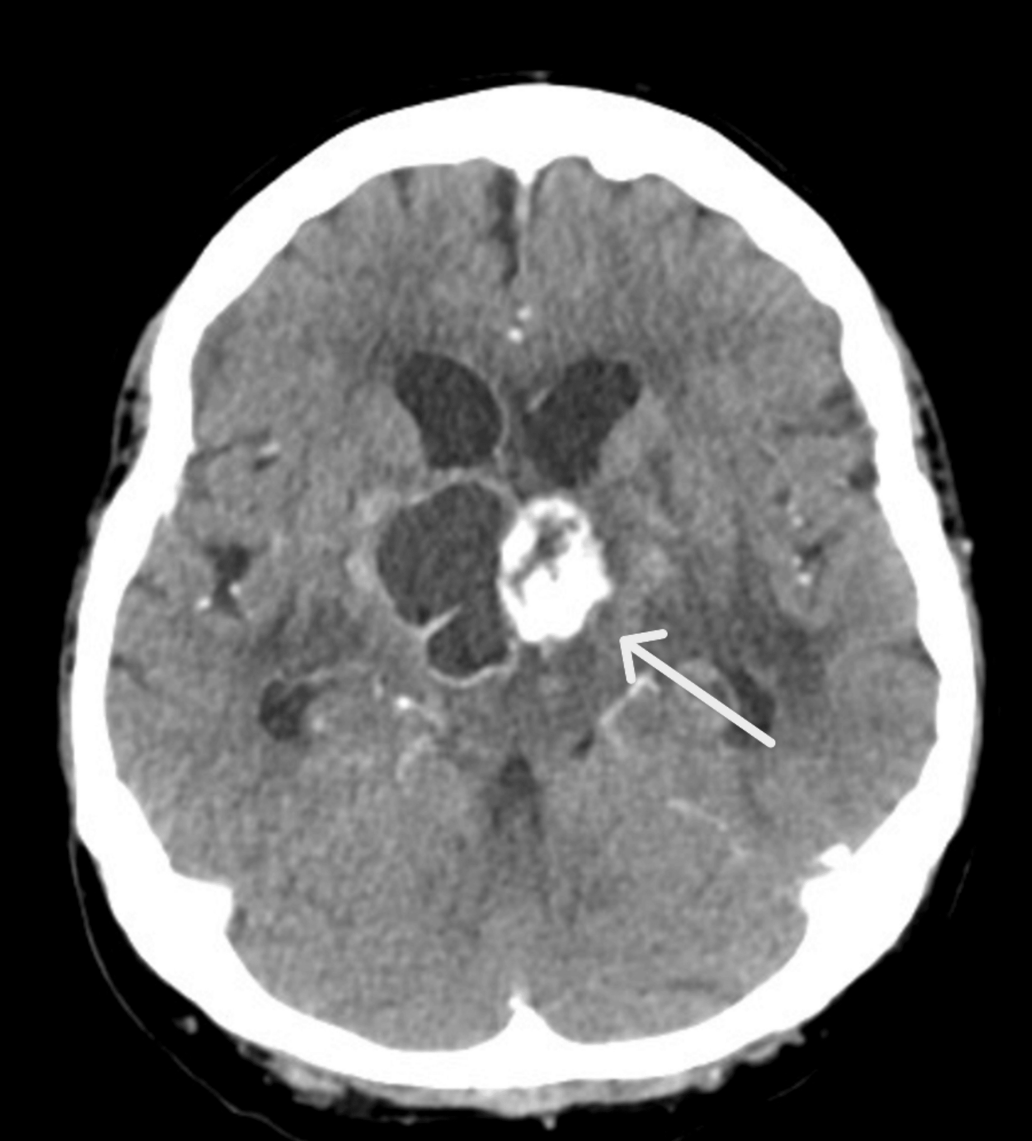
CT brain (axial view) with contrast performed on July 1, 2024 The known solid-cystic suprasellar-hypothalamic mass is larger in size, now measuring 3.8x3.5x2.9 cm. There is interval increase in the perilesional edema and local mass effect with compression of the floor of the third ventricle. New mild mass effect on the right frontal horn as well as interval increase in the size of the ventricles is noted, with prominence of bilateral temporal horns, suggesting early hydrocephalus. No acute intracranial hemorrhage or established territorial infarcts are noted.
